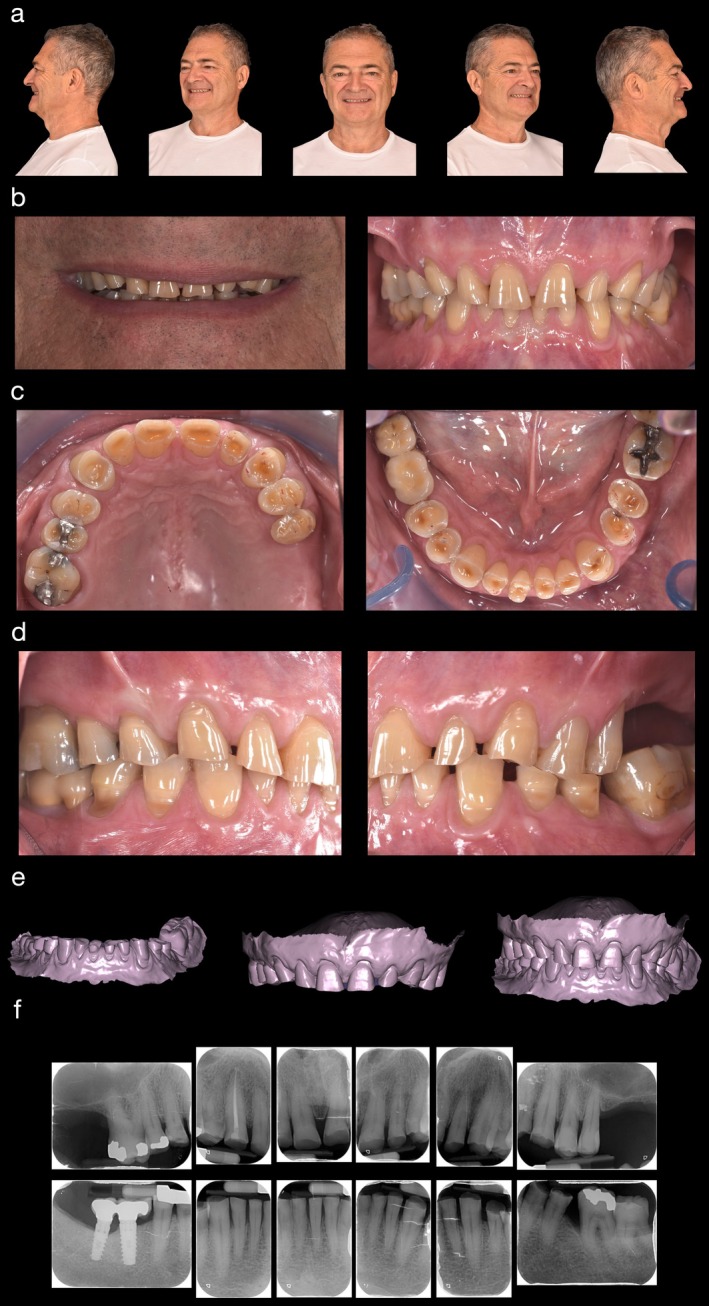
(a–f) Patient assessment and data collection: (a) extraoral images (smiling and not smiling), (b–d) smile detail and intraoral images (frontal, occlusal, lateral), (e) intraoral scan, (f) 2D radiographic images.
